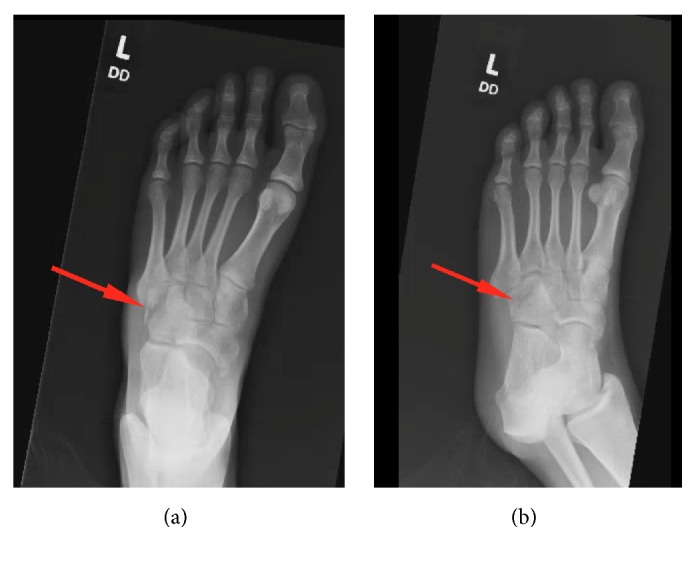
An X-ray of the left foot showed a commuted fracture of the cuboid bone with mildly displaced fragments, as well as moderate soft tissue edema (red arrows).
